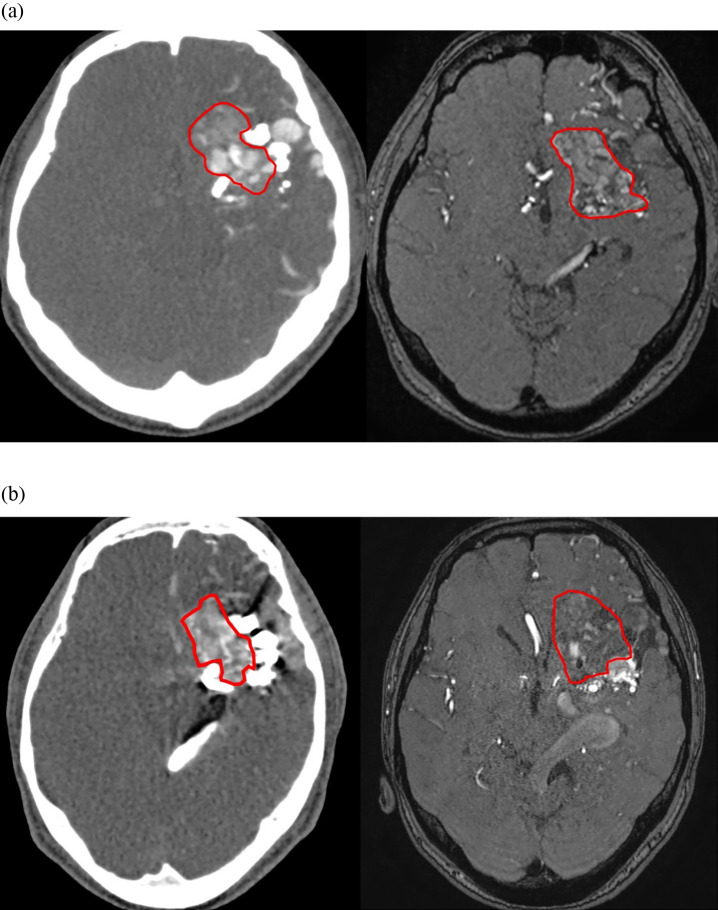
Fused four-dimensional computed tomography angiography imaging in case 1 reduced the clincal target volume compared with magnetic resonance angiography (MRA)-only fusion. a) In the first treatment, the planning target volumes for computed tomography angiography and magnetic resonance angiography were 20.54 and 32.99 cc, respectively. b) In the second treatment, the corresponding volumes were 15.94 and 30.54 cc, respectively. In both panels, the same slices are shown.
